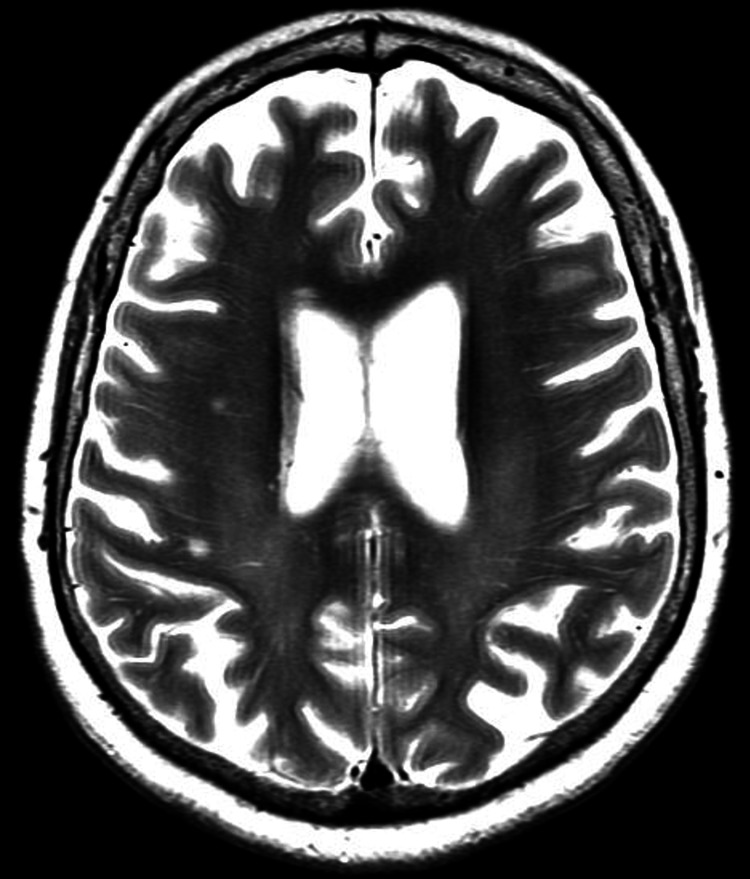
Axial view, T2-weighted MRI of the brain, with no noted parenchymal lesions or leptomeningeal enhancement.
